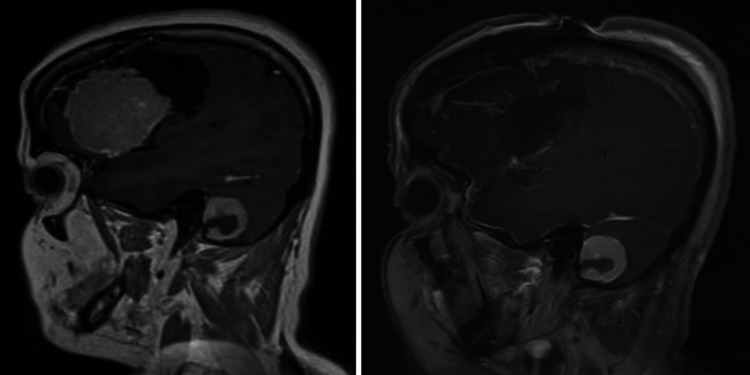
MRI brain T1 post-contrast, sagittal section, pre-operative (left) and post-operative (right) images. The lateral sphenoid-wing meningioma was completely resected as seen in post-operative scan on the right. Another homogenously enhancing lesion with a small hypo-intense central part, can be seen in the posterior fossa, which was not causing symptoms, was not resected.
